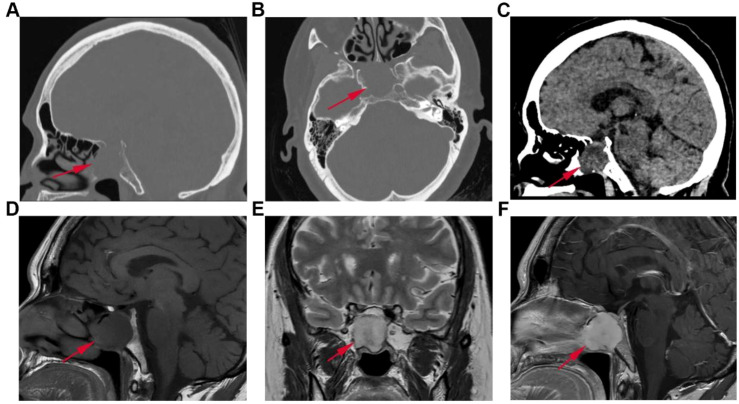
Preoperative imaging studies of the patient. (A–C) Cranial CT images: (A) Sagittal bone view. (B) Axial bone view. (C) Sagittal brain view. (D–F) Cranial MR images: (D) Sagittal T1-weighted image. (E) Coronal T2-weighted image. (F) Sagittal T1-weighted contrast-enhanced scan.
